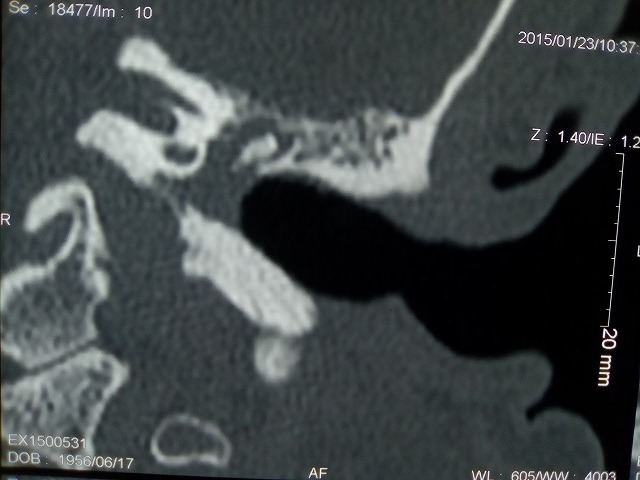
Coronal view of temporal CT showed complete opacification of the middle ear and mastoid indicating a soft tissue mass
